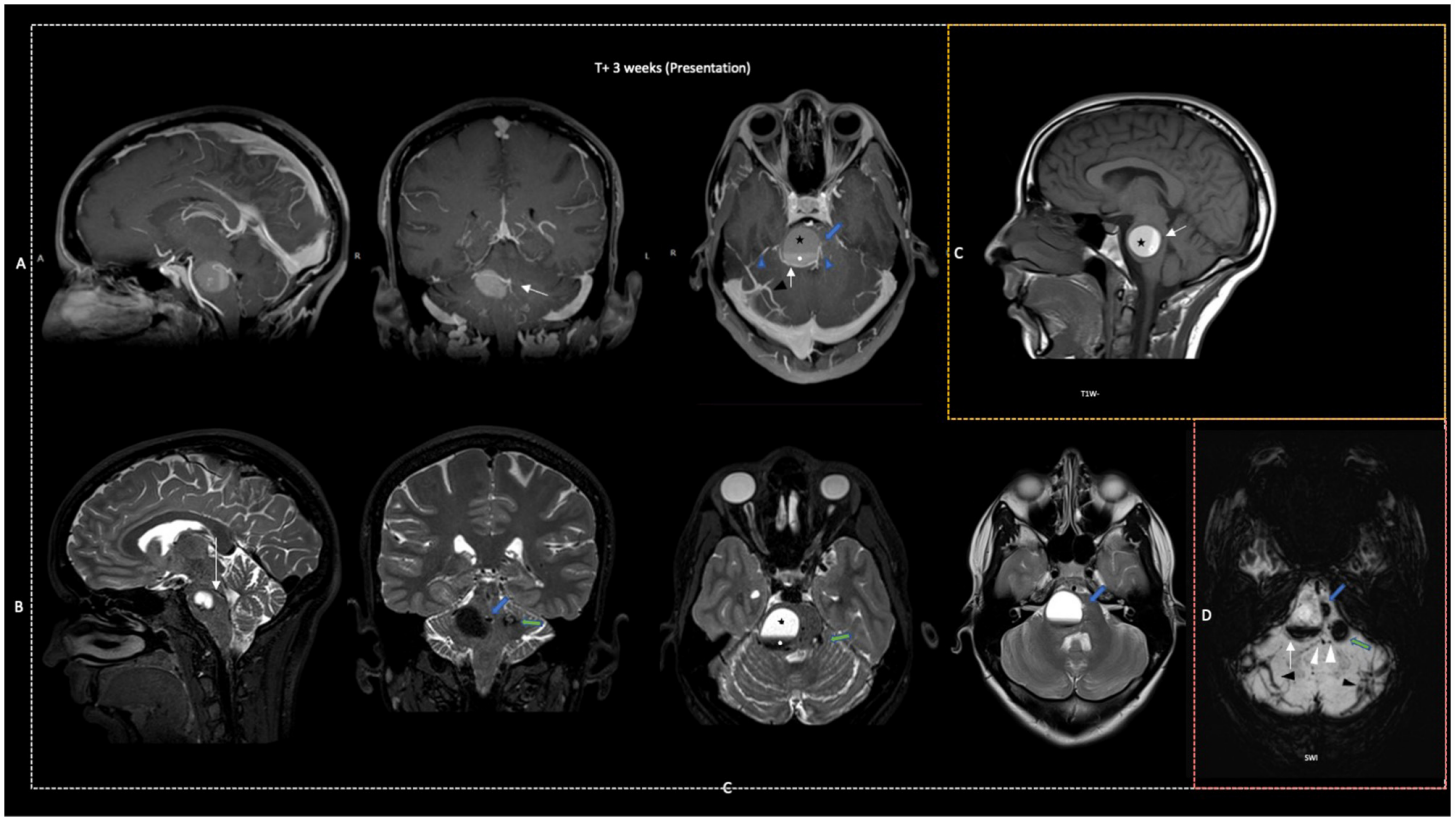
Pre-operative (first surgery). Sagittal, coronal, and axial MRI: (A) T1W + Gad; (B) T2W; sagittal only: (C) TW1 and axial only: (D) SWI. Showing partly hyperacute hematoma (black star) of T1W isointense and T2W hyperintense signal. Early subacute hematoma portion (white star) of T1W hyperintense and T2W hypointense signal. Associated: Two CCMs (target/culprit—blue arrow; incidental contralateral cerebellar peduncle—green arrow) seen as blooming artifacts on SWI, popcorn appearance of mixed signal intensity on T2W, outline against the medial aspect of hematoma cavity on T1W-DVA with a retrospectively determined smaller caliber of its main draining vein (white arrow) seen on T1W + Gad filling, serpiginous flow void on T2W and SWI. Draining *via* multiple collector veins on T1W+ (blue arrowheads) into caput medusae appearance of transcerebellar veins on SWI (black arrowhead). Prominent channels can be seen connecting both CCMs likely due to high pressures (white arrowheads on SWI).
